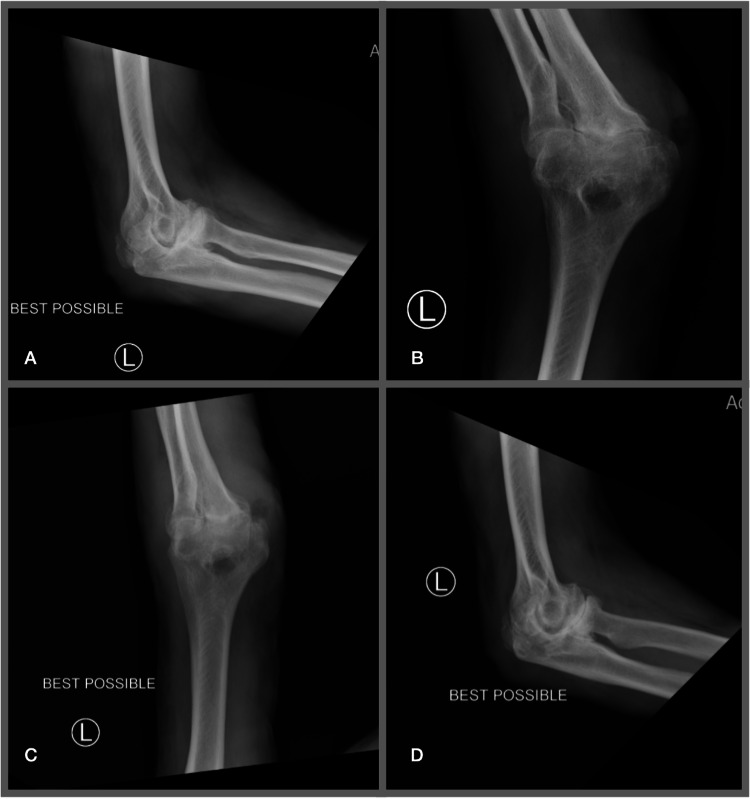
Left elbow X-ray (multiple views) A: Anterior-posterior view B: Anterior-posterior oblique with externally rotated arm C: Lateral view extended arm D: Lateral view flexed arm
